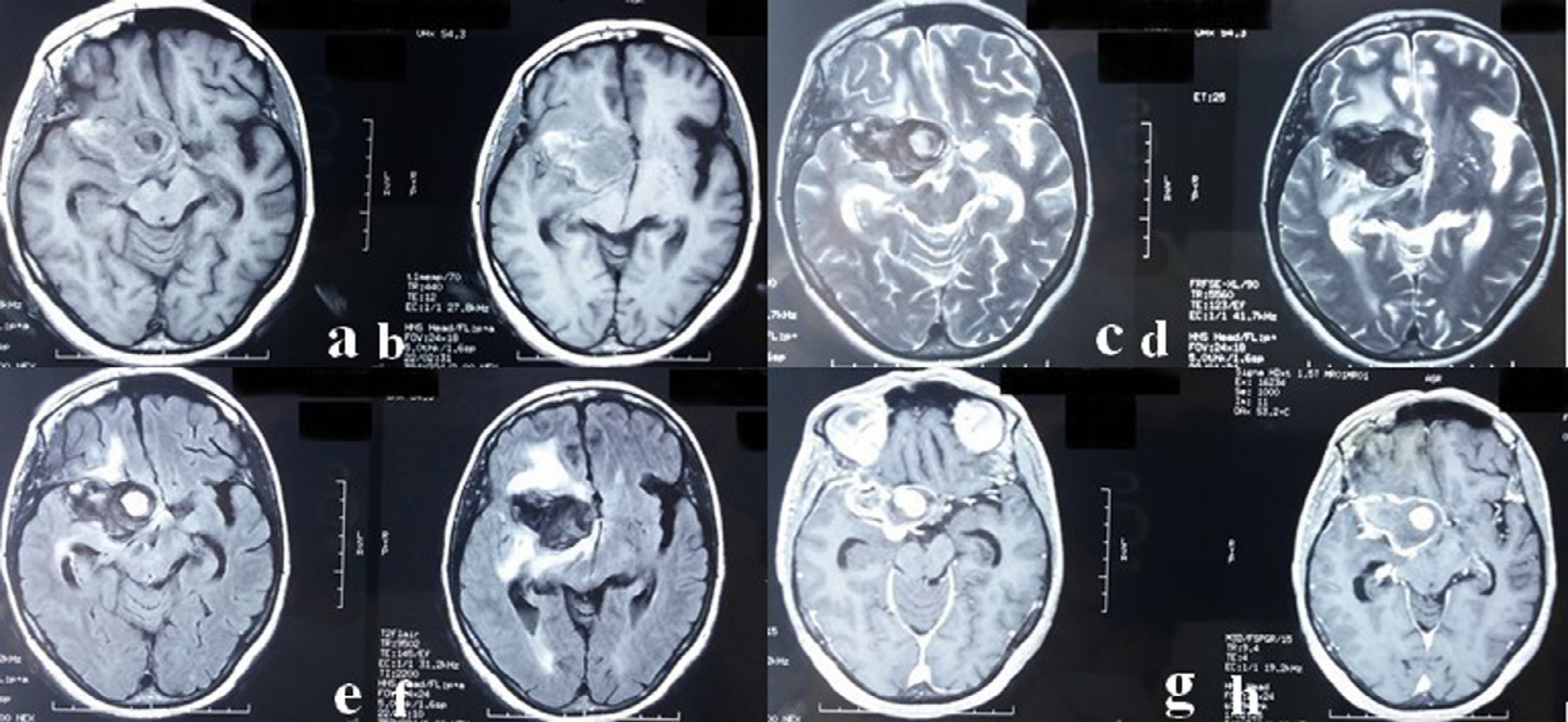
Axial brain magnetic resonance imaging (MRI) showing a suprasellar lesion measuring 45 × 4 × 39 in diameter lateralized to the right side with heterogeneous hyposignal on T1 weighted image (A,B) and hyposignal on T2 weighted image (C,D). The lesion appeared also in heterogeneous hypersignal on the Fluid attenuated inversion recovery (FLAIR) sequence (E,F) with a significant surrounding perilesional edematous reaction resulting in a mass effect on the optic chiasm and the third ventricle as well as an active biventricular hydrocephalus. Note the peripheral heterogeneous enhancement similar to the arterial network communicating with the internal cavernous carotid artery after Gadolinium administration (G,H) suggesting a partial thrombosed aneurysm.
